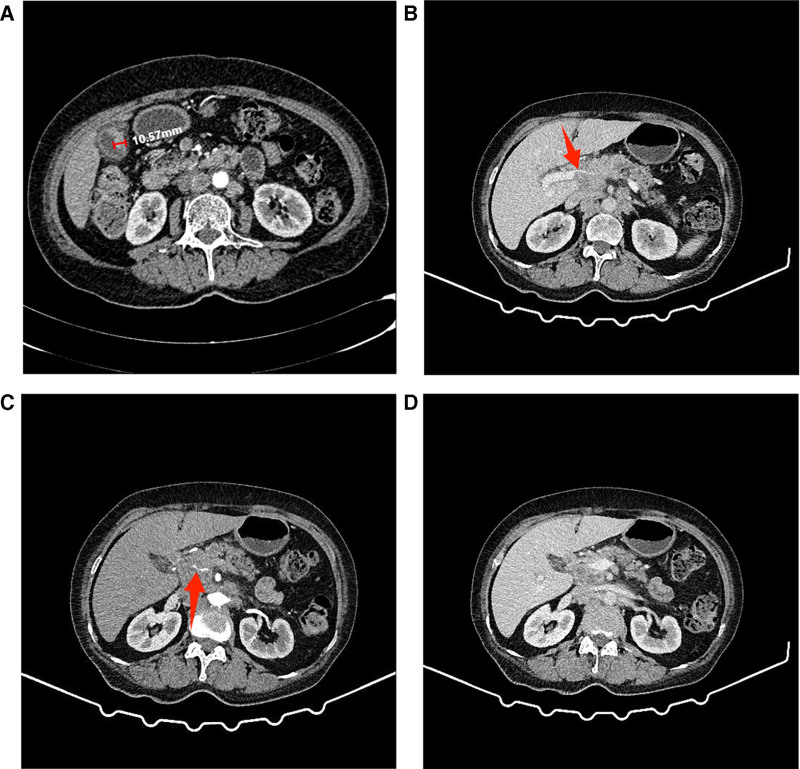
Initial CT findings. (A) Irregular focal wall thickening at the gallbladder fundus, measuring approximately 10.57 mm in thickness. (B) Invasion of the portal vein (indicated by a red arrow). (C) Invasion of the hepatic artery (indicated by a red arrow). (D) Multiple enlarged lymph nodes in the porta hepatis and retroperitoneum.
